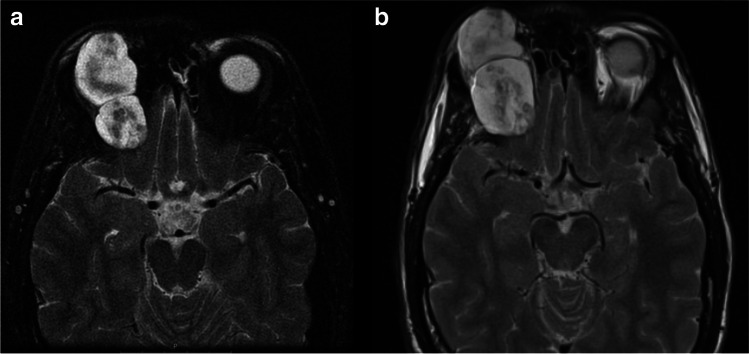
a) MRI 5 years after initial diagnosis of a large orbital schwannoma in a patient who had refused surgery (Patient 8), b) MRI of the same patient 10 years later showing further growth during the observation period
